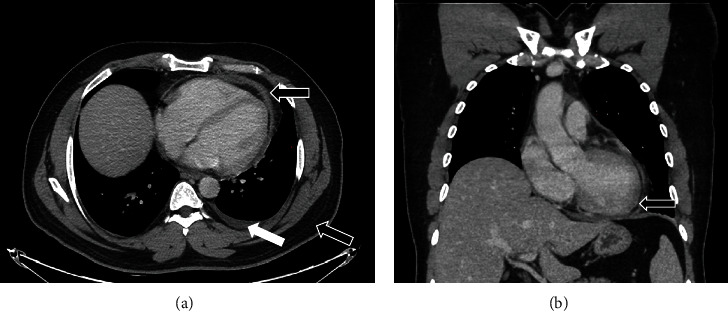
(a) The axial image and (b) the coronal image of the CT scan of the chest post administration of IV contrast showing diffuse pericardial thickening and pericardial fat stranding (black arrow) with small left pleural effusion (white arrow).
